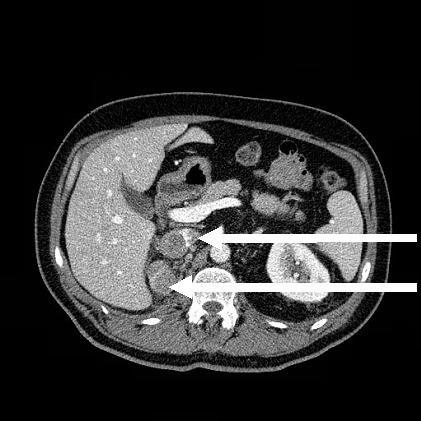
Preoperative CT scan: upper arrow pointing to the inferior vena cava with tumor thrombus, the arrow bellow pointing to the metastasis.
